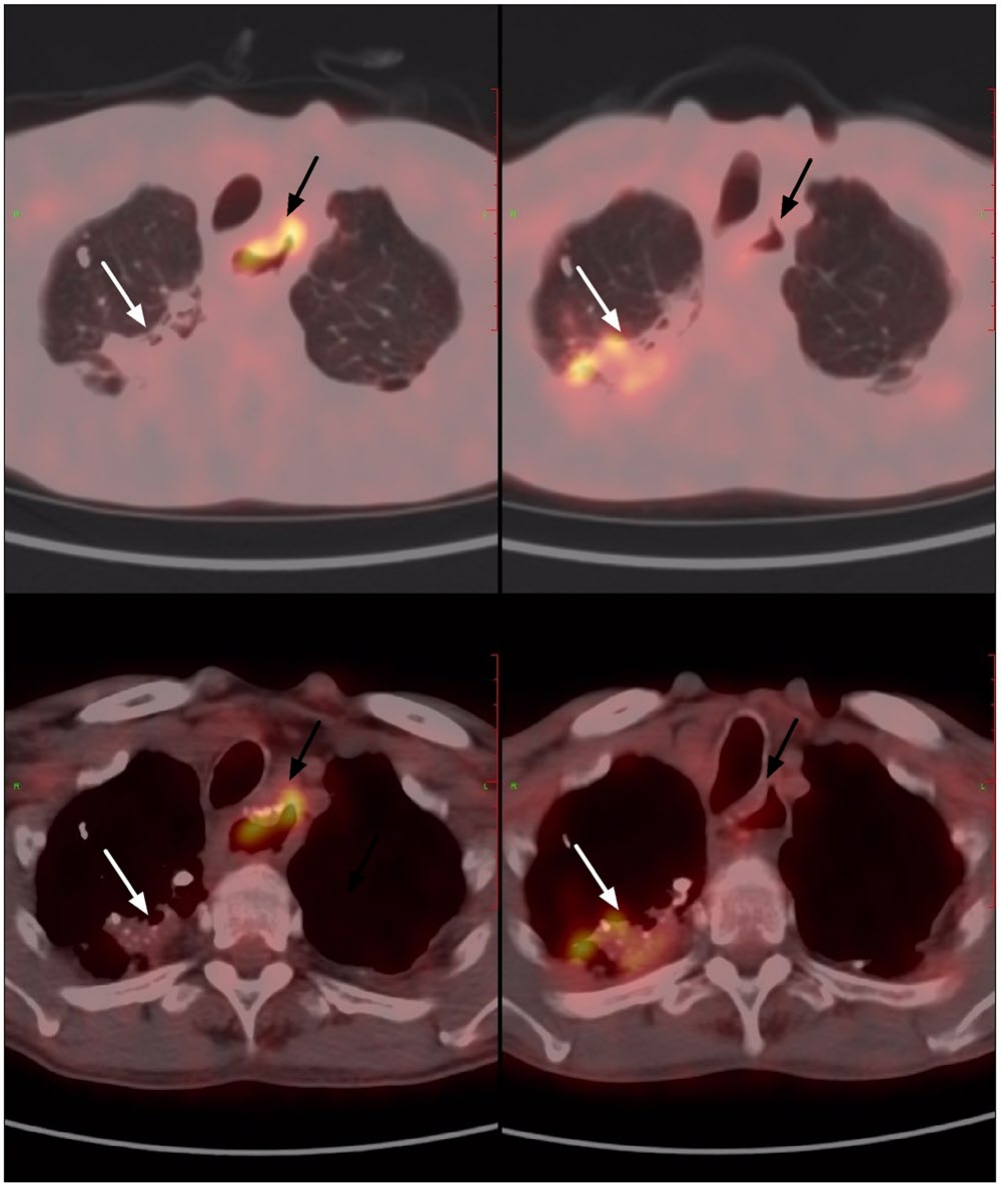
A 54-year-old man with history of esophageal cancer. Old tuberculosis lesions were seen at the apex of right lung (white arrow), and the FDG hypermetabolism anastomosis after esophageal cancer surgery (black arrow). 1 year later, the PET/CT follow-up showed that the volume of old tuberculosis increased, and the SUVmax increased from 1.6 to 4.0.
